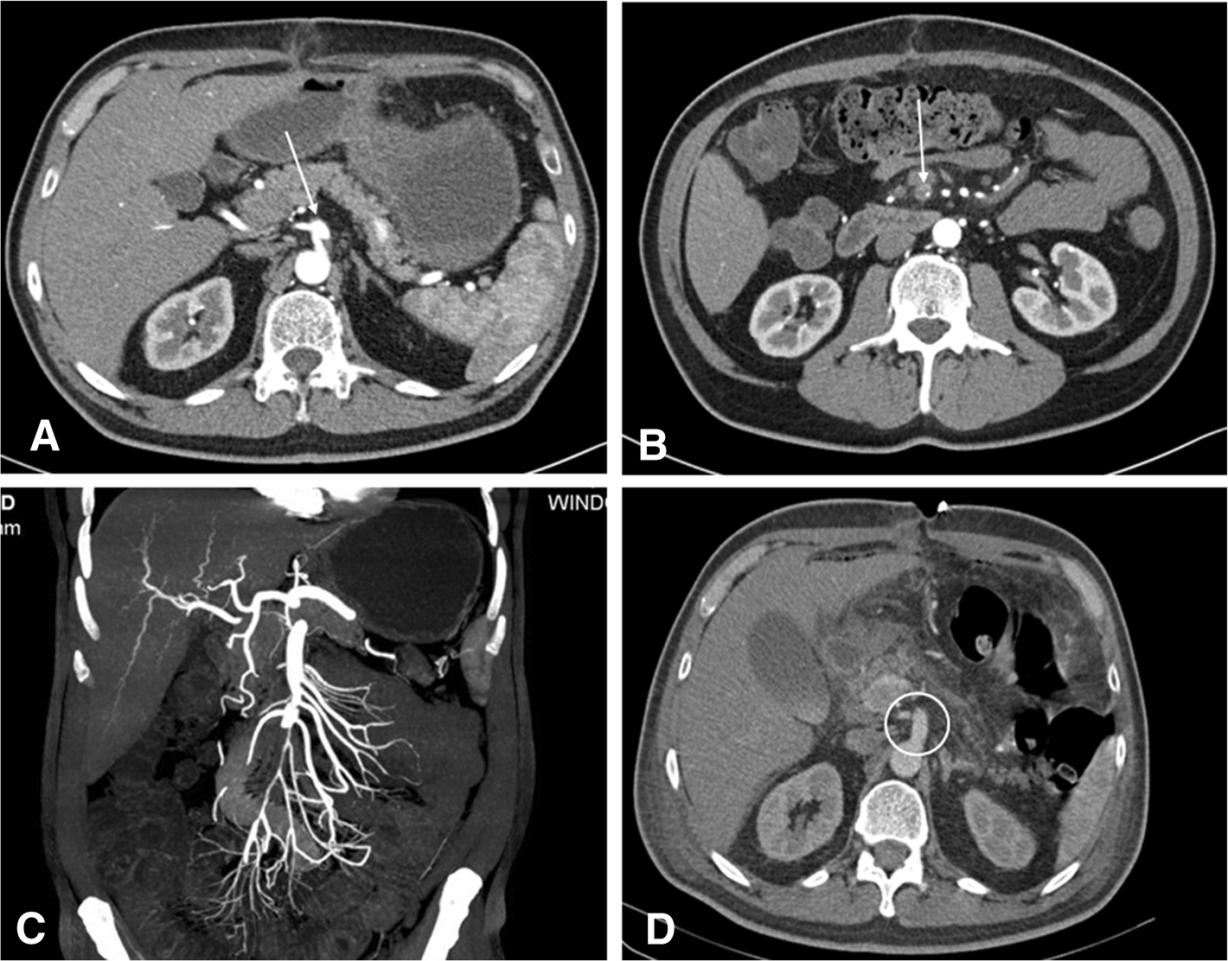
Representative CT scan findings. A: shown is the entry of the dissection at the proximal SMA. An abnormal origin of the right hepatic artery from the proximal SMA can be seen as an anatomical variant. B: An embolism of a distal branch of the SMA is shown. C: Reconstruction of the CT scan after admission. Almost complete decline of intestinal perfusion of the right abdominal side could be observed. D: findings of the control CT scan 5 days after operation. No residual membrane could be observed, normal perfusion of the SMA and the right hepatic artery.
